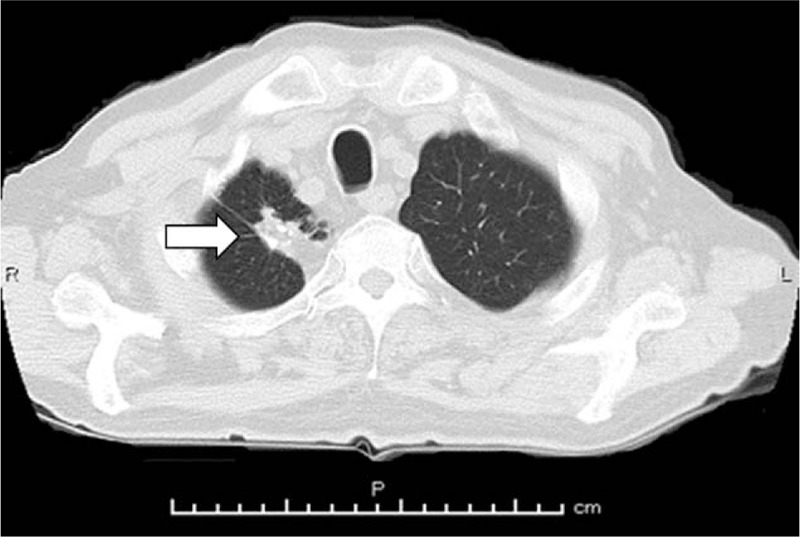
Computed tomography revealing calcified nodule (white arrow) in the right upper lobe of the lung, long before or, at least since, the diagnosis of prostate cancer.
